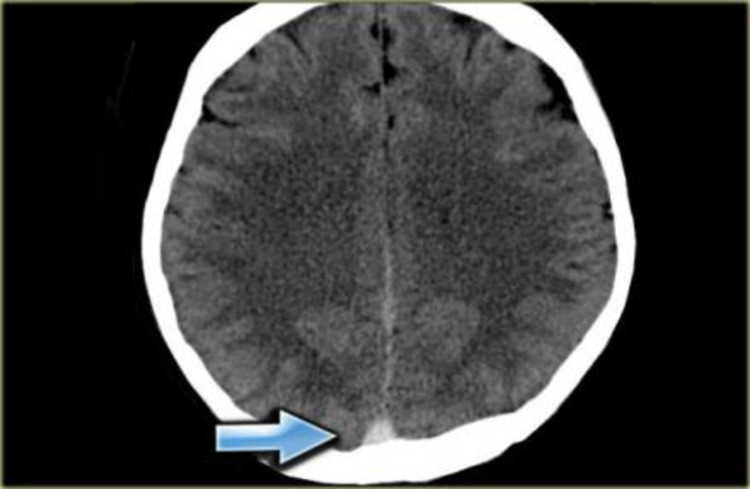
CECT brain showing CVST (arrowhead) CECT: contrast-enhanced computed tomography; CVST: cerebral venous sinus thrombosis
